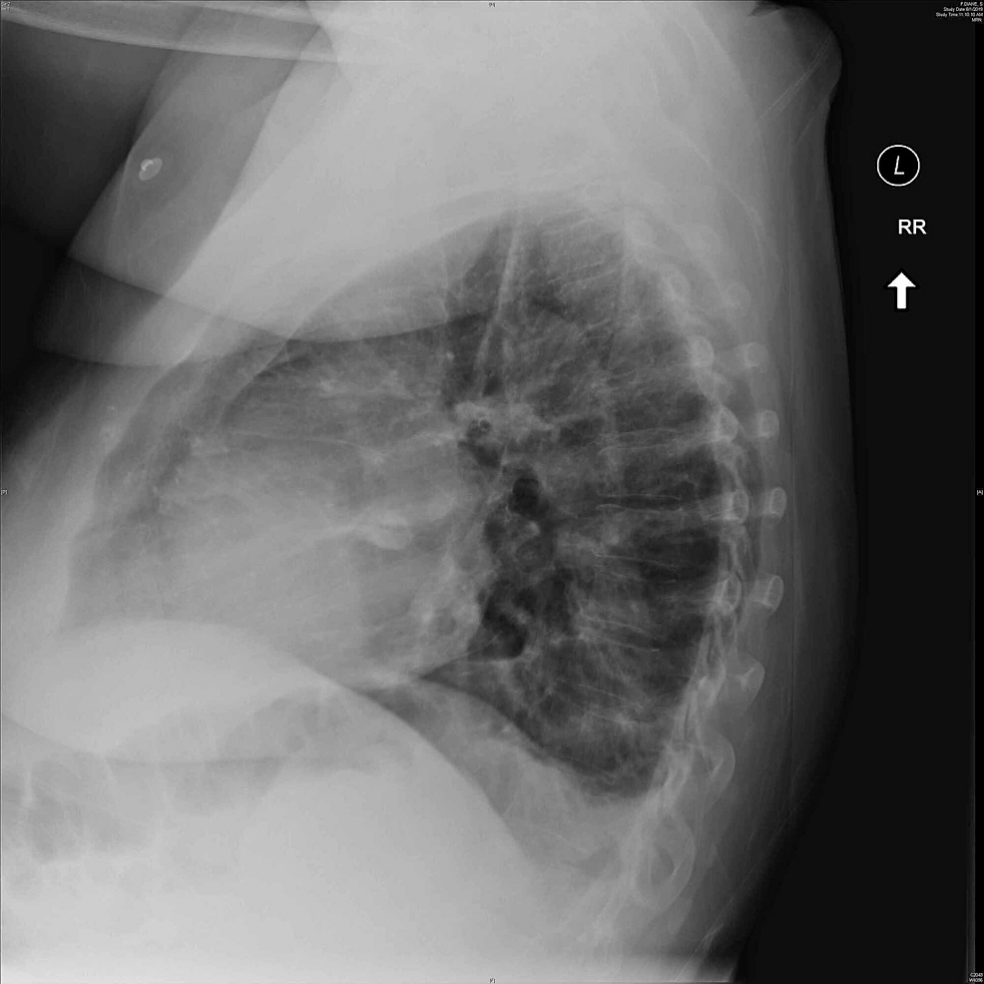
Subsequent lateral chest radiograph revealed no opacity.
